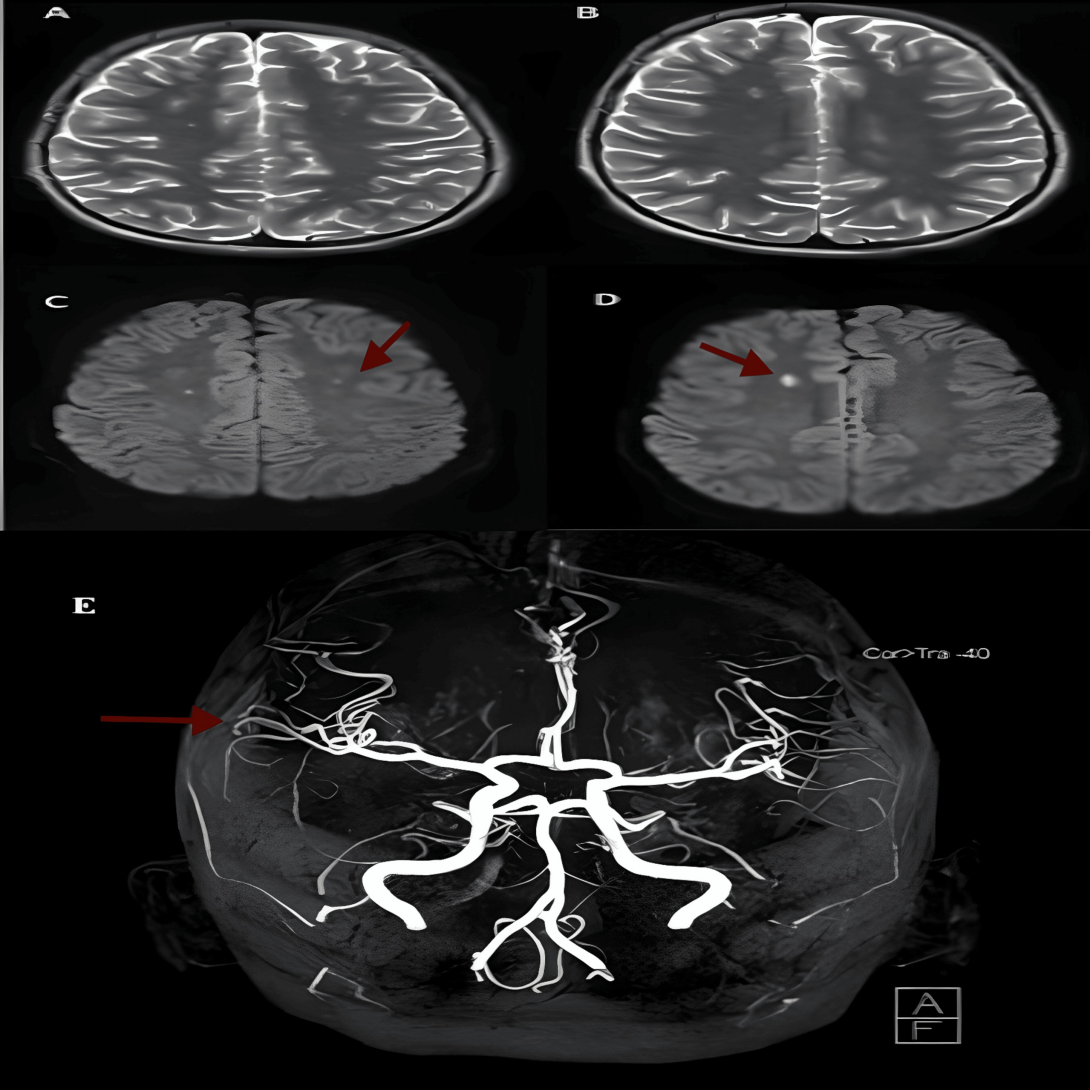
(A-D) MRI brain axial T2 sequences show high signal intensity foci involving both coronal radiata (bilateral centrum semiovale) (A) and the right frontal lobe (B), with corresponding restricted diffusion on axial diffusion-weighted images (DWI) (C, D), suggestive of neuropsychiatric SLE (arrows indicate the findings); (E) 3D TOF MR angiography in coronal reconstruction of the cerebral and cerebellar arteries demonstrates a normal appearance of the major arteries and their branches (arrow for reference). 3D TOF MR, three-dimensional time-of-flight magnetic resonance; SLE, systemic lupus erythematosus
